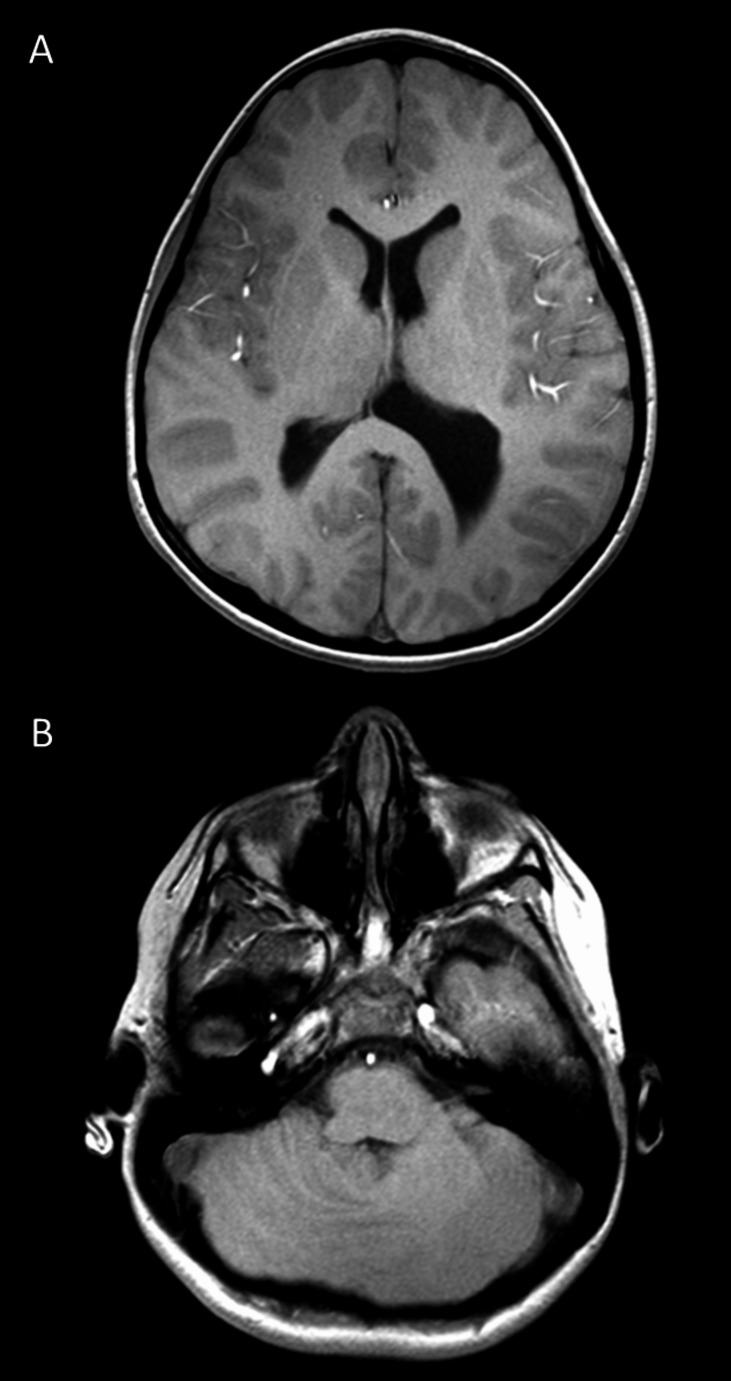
T1-weighted axial 3T MRI scan at the age of 8 years showing left ventriculomegaly (A) and fusion of the cerebellar hemispheres (B)
